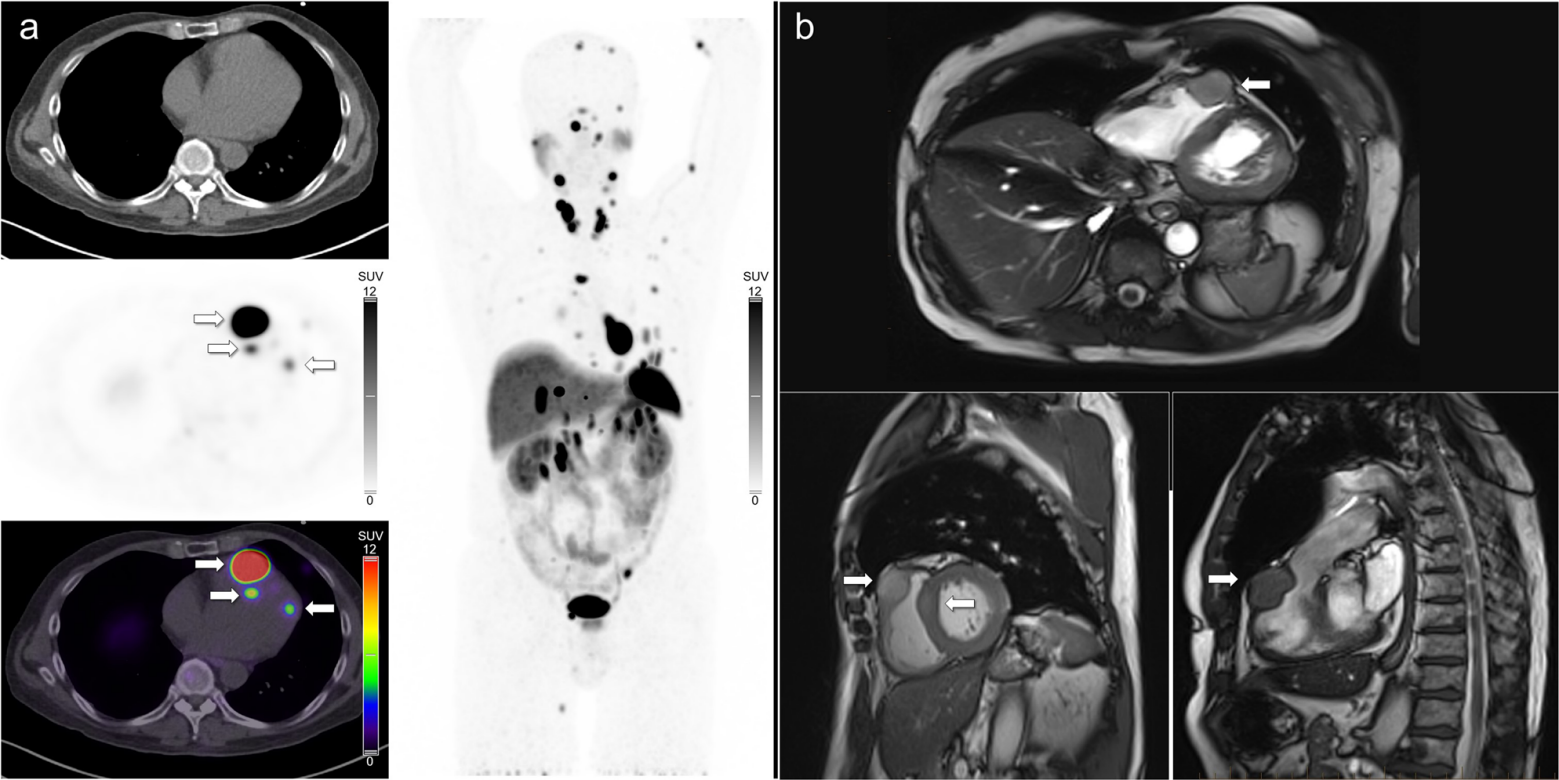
(A) [^68^Ga]Ga-DOTATATE PET/CT images in a patient with metastatic pulmonary NEN demonstrating a large intensely avid metastasis in the right ventricular outflow tract, with two other smaller avid metastases in the left ventricle. (B) CMR images (multiplanar steady-state free precession sequences) better characterize the size and functional significance of the right ventricular outflow metastasis just proximal to the pulmonary valve with luminal narrowing. One of the left ventricular lesions is subtly visible on the two-chamber view. The patient proceeded to surgical resection of the right ventricular outflow tract lesion prior to PRRT
